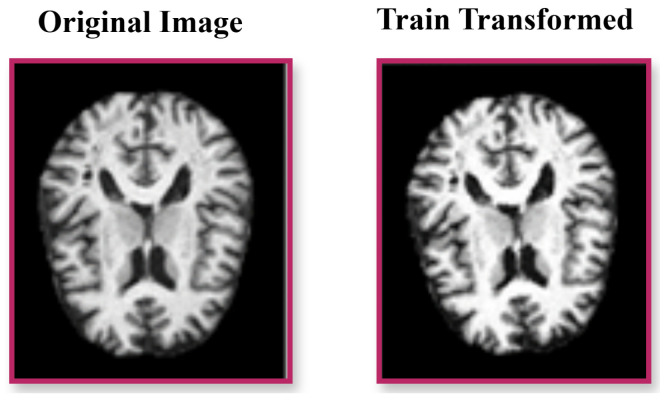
Original T1-weighted MRI and its augmented transformations.
